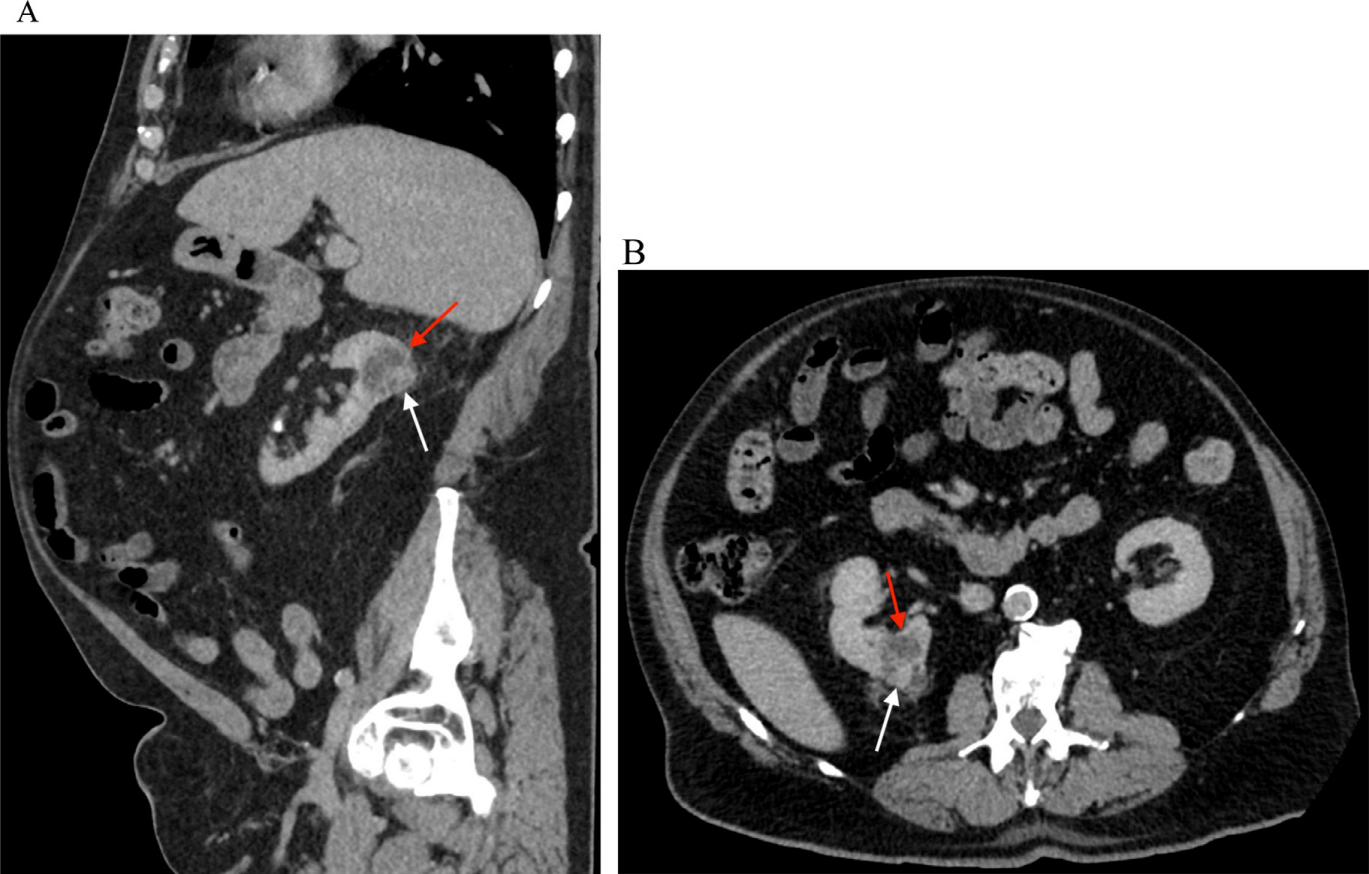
A contrast-enhanced CT of the abdomen and pelvis. (A) Sagittal and (B) axial CT images demonstrate a complex cystic mass in the posterior upper right kidney measuring up to 3.8 × 2.5 × 2.8 cm (red arrow) containing numerous thin and mildly thickened septations and a solid enhancing nodular component along the posterior aspect measuring up to 8 mm in thickness (white arrow).
